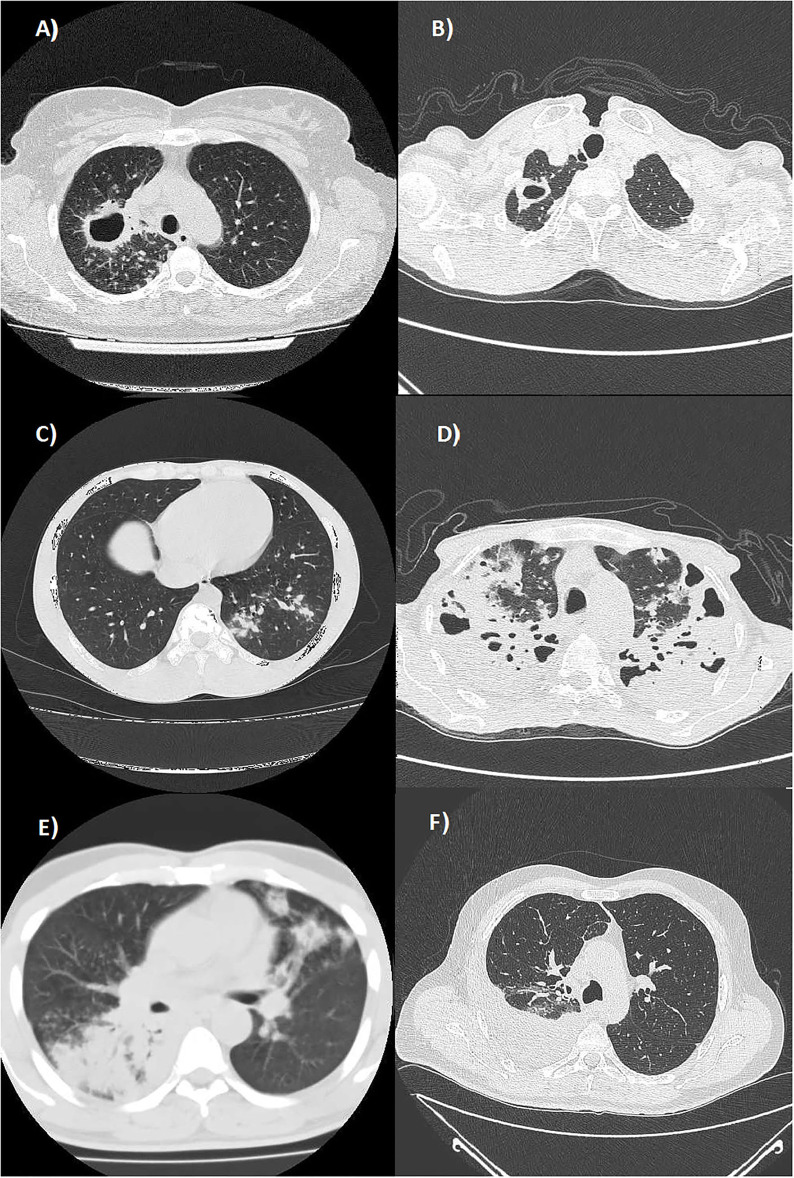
Axial Chest CT scans (lung window) showing: (A) multiple well-defined nodules diffusely distributed in the upper lobes and a cavitated lesion with thickened walls in the posterior segment of the right upper lobe (productive form with typical location); (B) a cavitated lesion with thickened walls in the apical segment of the right upper lobe (productive form with typical location); (C) a nodular pattern in the posterior segment of the left lower lobe (productive form with typical location); (D) a multifocal bilateral tubercular pneumonia in the upper lobes (exudative form with atypical locations); (E) multifocal tubercular pneumonia affecting the superior segment of the right lower lobe and the lingula (exudative form with atypical locations); and (F) a right tuberculous pleural effusion (exudative form with atypical locations).
